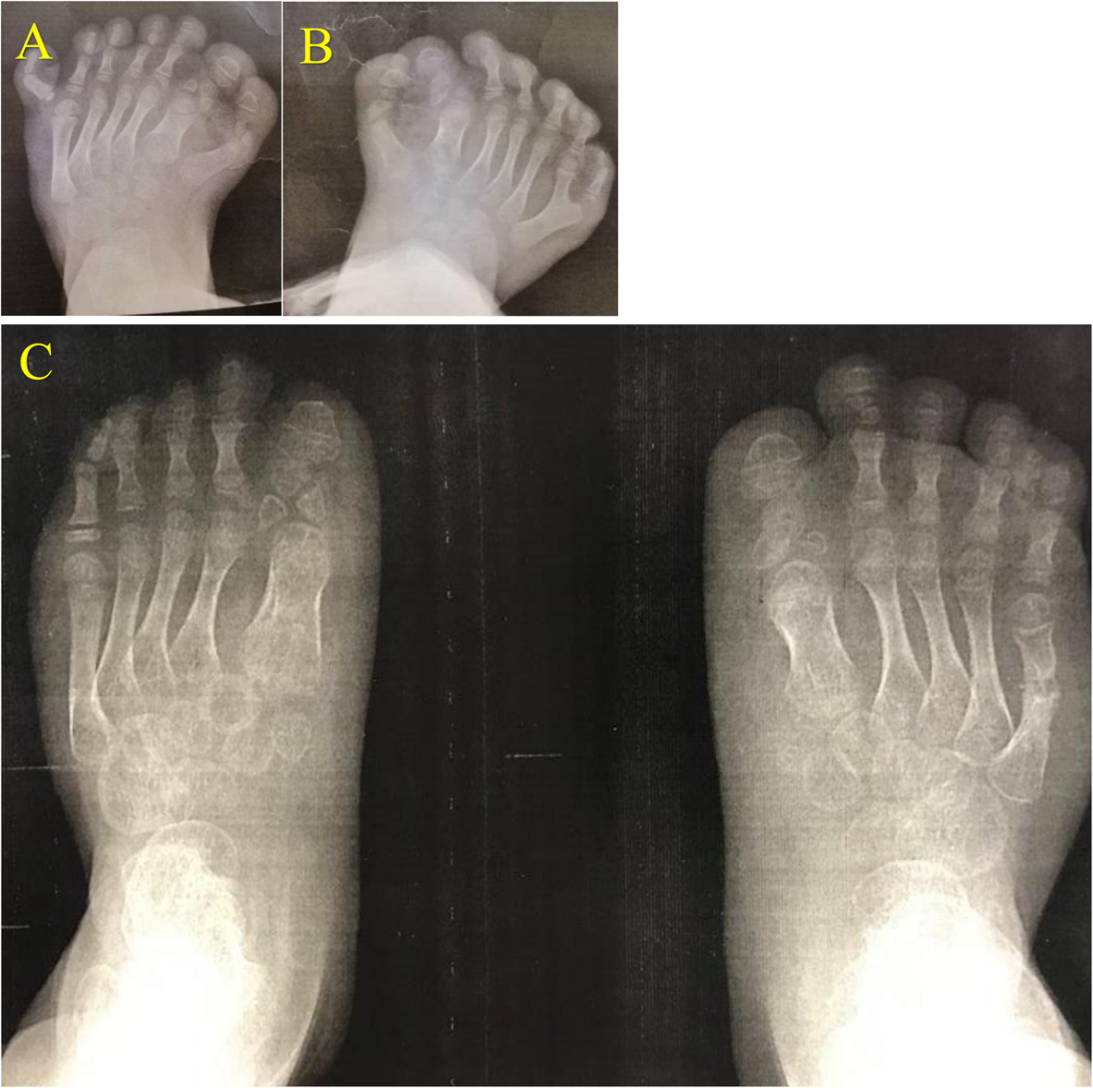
left (a) and right (b) foot anteroposterior radiographs of the patient showing bilateral polydactyly before and after (c) surgery
